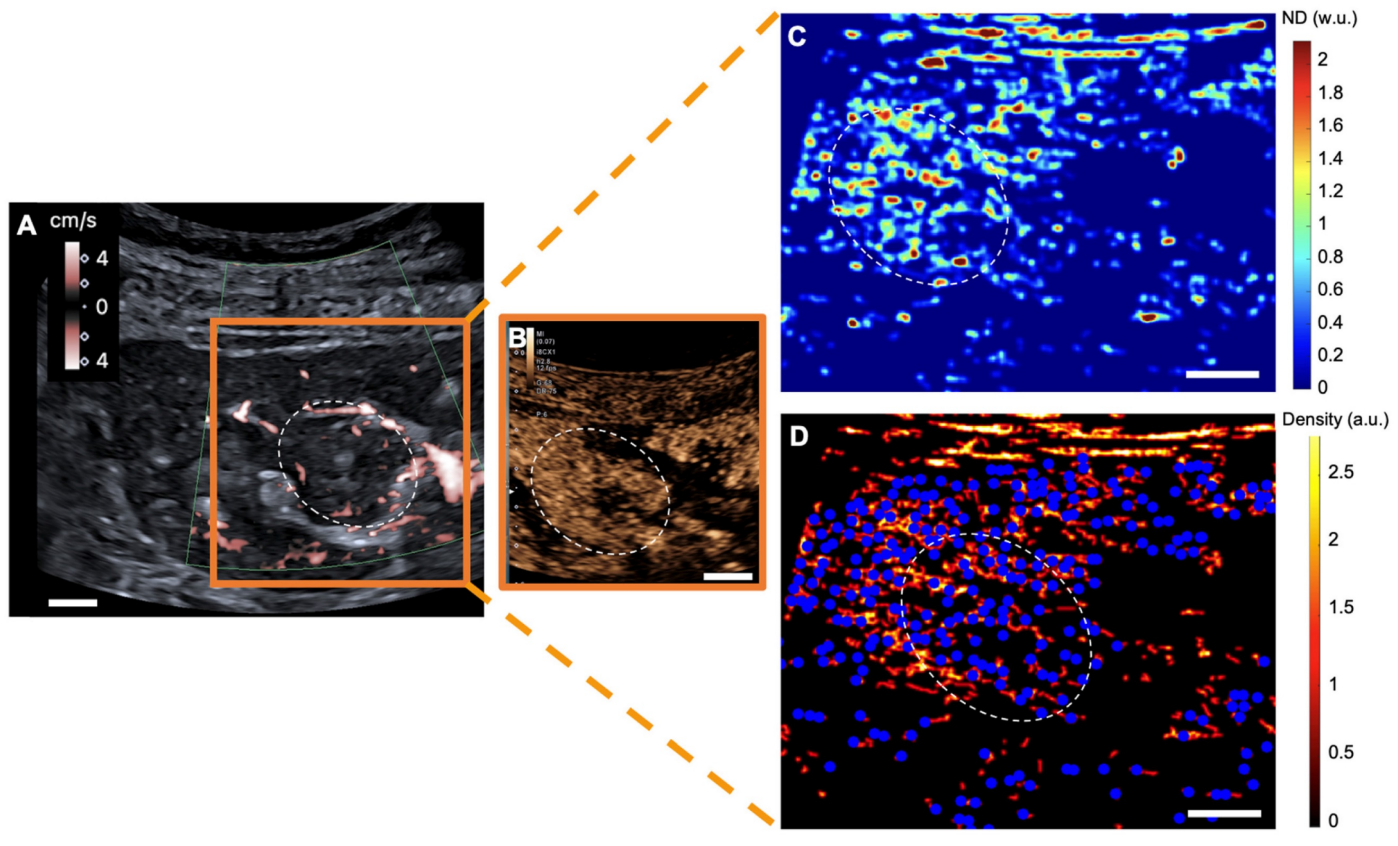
sULM of hypertrophy of the column of Bertin (patient 7). A & B. Superb microvascular imaging Doppler image (A) and CEUS (B) showing an endophytic renal pseudotumor (hypertrophy of column of Bertin) (*white-dotted area*). C & D show normalized distance metrics. (C) This metric enhances glomerular behavior, highlighting the detected glomeruli in blue points on the density map. (D) Note the presence of glomerular paths in the renal cortex and the pseudotumor. (The traces projected on the grid are displayed in red on the image. The colorbar corresponds to the count of the number of bubbles per pixel. Scale bars indicate 10 mm. *ND= normalized distance; w.u.= without units; a.u. = arbitrary units*.
